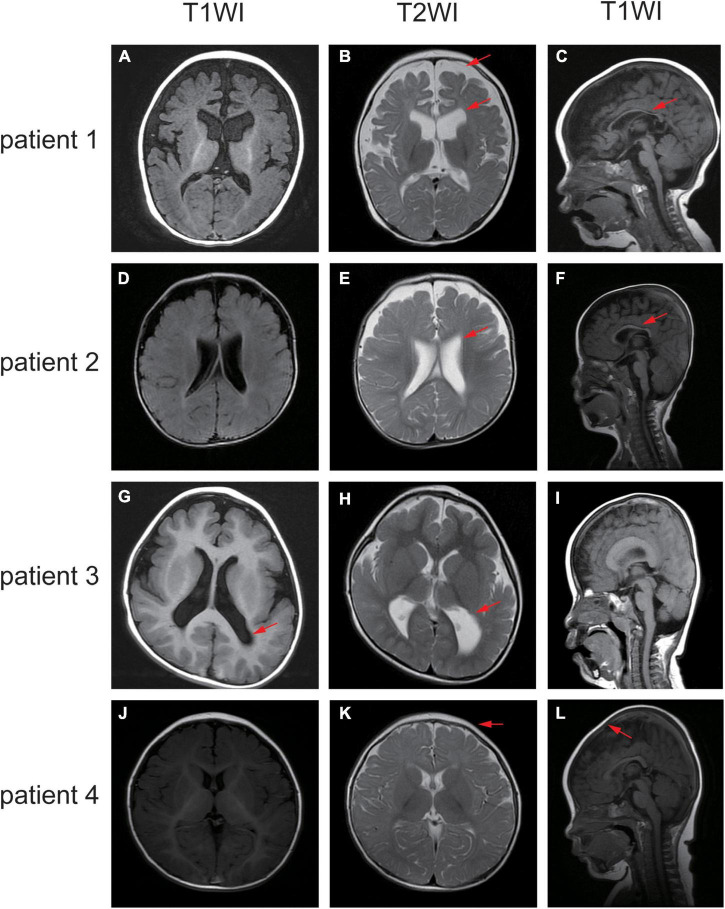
Different sequence of the brain MRI of the affected individuals. (A–C) MRI of patient one demonstrated widened extracerebral interspace, dilatation of the bilateral lateral ventricles and dysgenesis of the corpus callosum. (D–F) MRI of patient two showed bilateral ventriculomegaly and thin corpus callosum. (G–I) MRI of patient three revealed the posterior horn of bilateral ventricle and the trigone were enlarged bilaterally. (J–L) MRI of patient four elucidated sharp frontal anterior skull and decreased anteroposterior diameter of the skull. The red arrows indicate the positions of the lesions.
